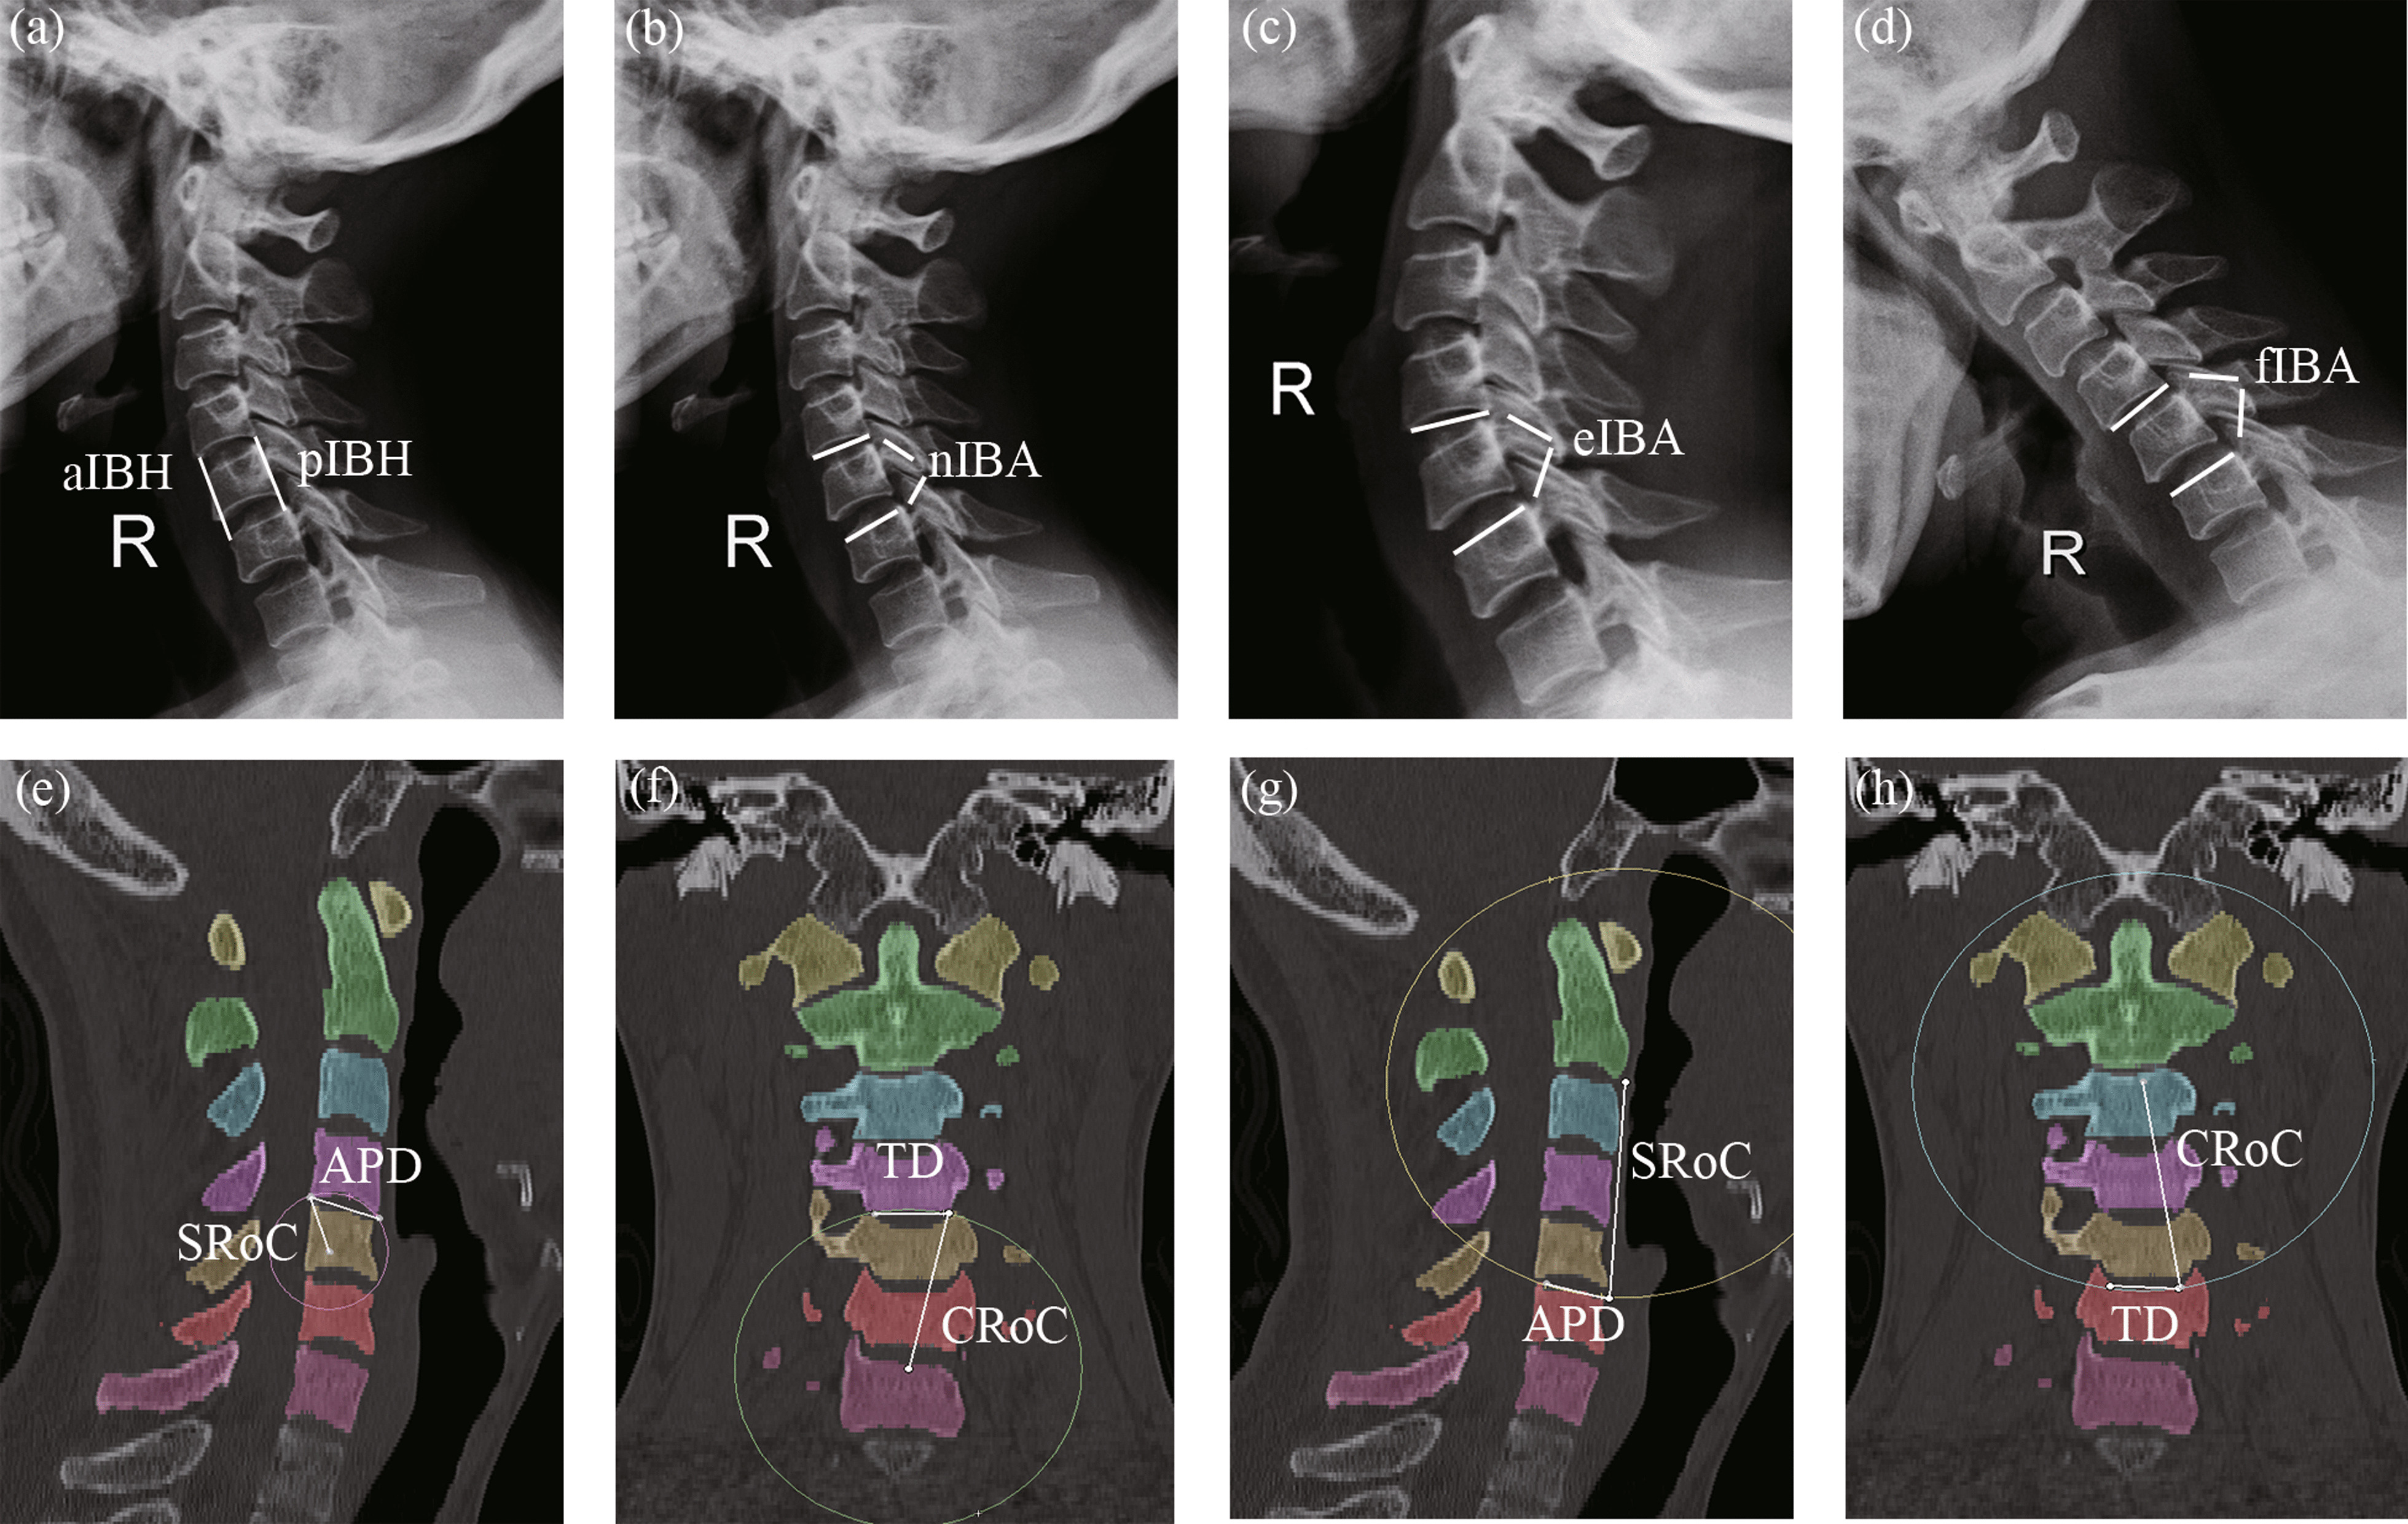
Measurement of parameters of C4–6 in the X-ray and CT images. a Measurement of anterior intervertebral body height (aIBH) and posterior intervertebral body height (pIBH); b measurement of lateral neutral intervertebral body angle (nIBA); c measurement of extension IBA (eIBA); d measurement of flexion IB A (fIBA); e Measurement of sagittal anteroposterior diameter (APD) and the middle sagittal radius of curvature (SRoC) of C4 lower endplate; f Measurement of coronal transverse diameter (TD) and the middle coronal radius of curvature (CRoC) of C4 lower endplate; g Measurement of APD and SRoC of C6 upper endplate; h Measurement of TD and CRoC of C6 upper endplate
